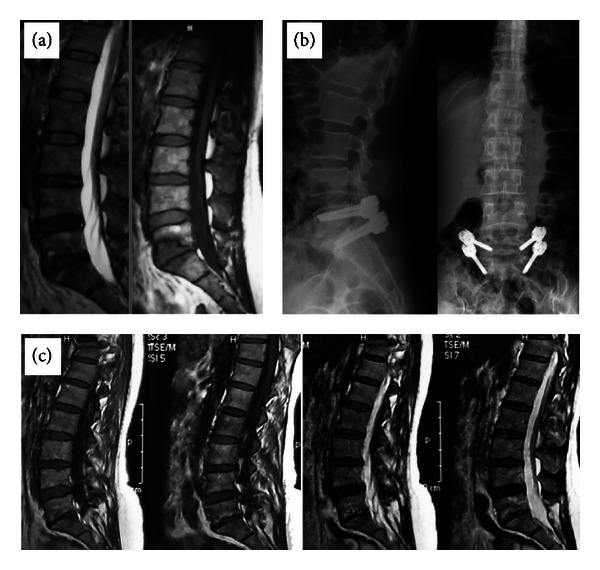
A 43-year-old female patient complained of severe back pain, particularly when standing or walking. (a) T1- and T2-weighted images showing hypointense corpus changes in upper and lower endplates. (b) Dynamic stabilization carried out with Safinaz screws. (c) T1- and T2-weighted MR images showing degenerative changes that shifted to Modic type 3, 2 years later.
